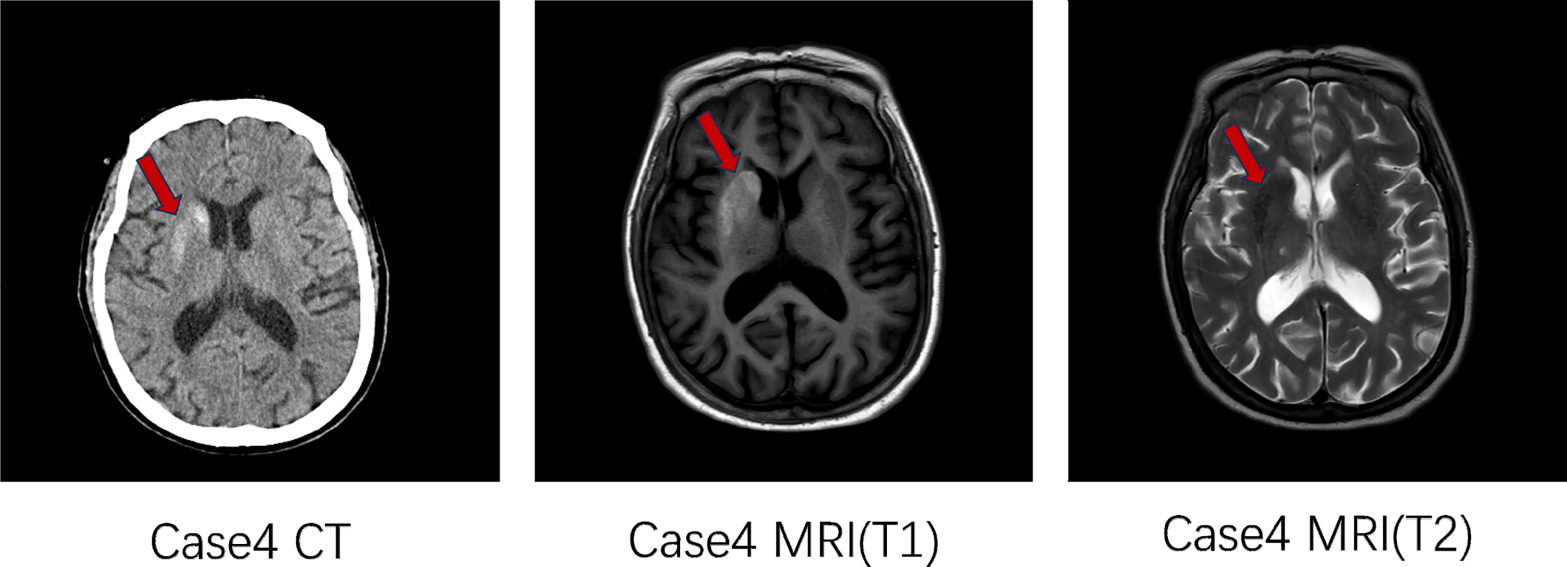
Imaging manifestation of case 4. CT, computed tomography; MRI, magnetic resonance imaging.
